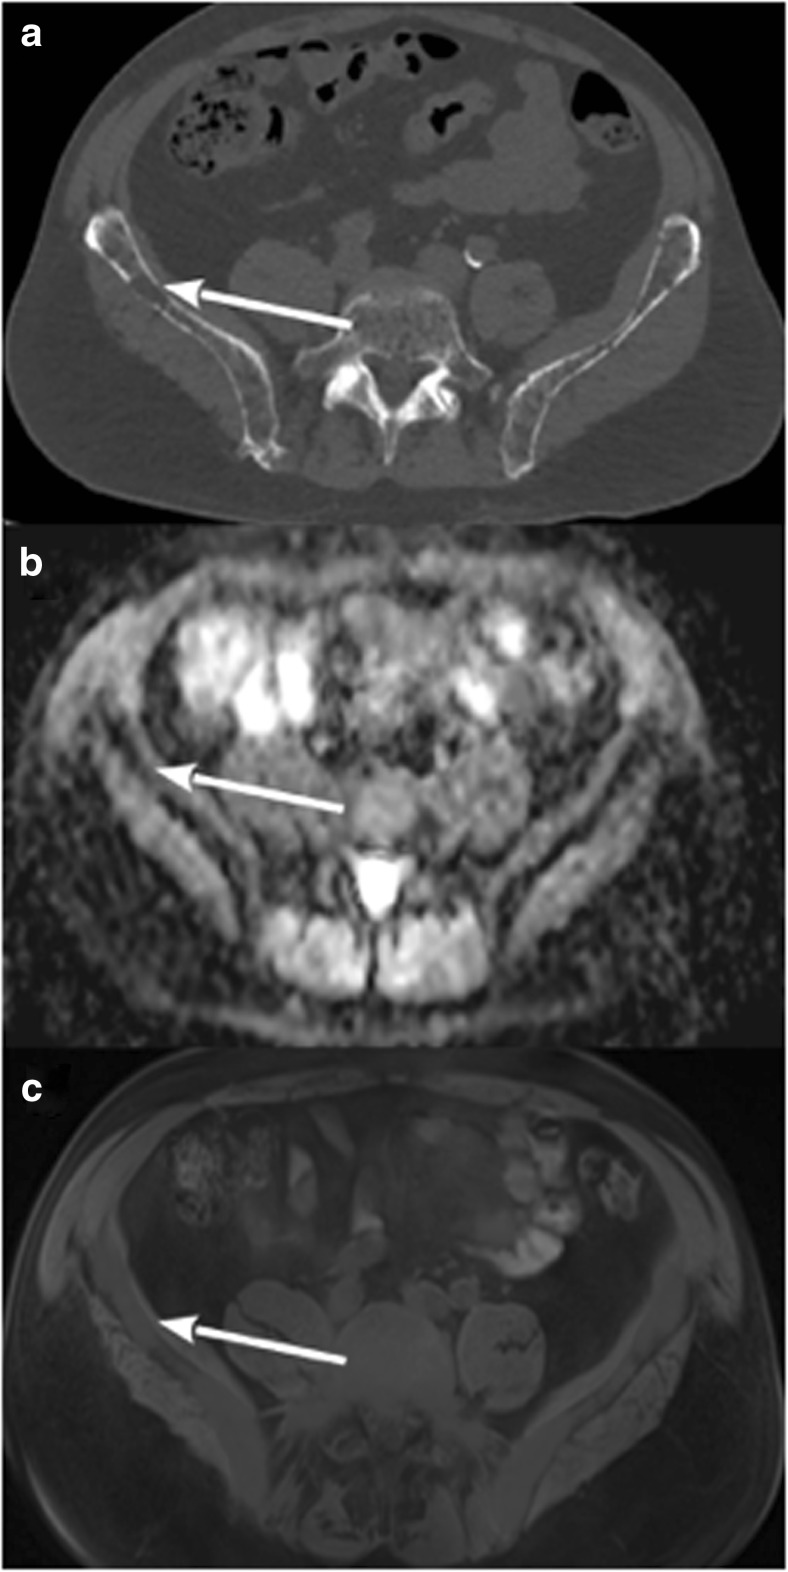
a WB-LDCT showing lytic bone lesion (white arrow) with negative densitometry of − 46 HU. b ADC map with ADC value of the lesion of 346 × 10^−6^ mm^2^/s. c Fused PET/MRI image showing normal FDG uptake of the area with SUVmax of 1.09
